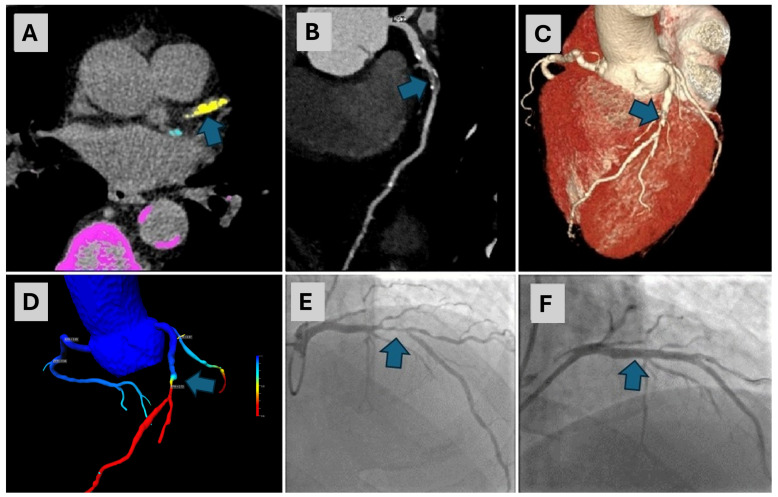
An 80-year-old male with a CAC score of 868.5, exhibiting prominent calcifications in the LAD artery ((A)—arrow), underwent CCTA. The CCTA revealed severe stenosis in the mid-segment of the LAD artery ((B)—arrow, (C)—arrow). A cFFR test confirmed a significant stenosis in the same segment ((D)—arrow), with a cFFR value of less than 0.7. Consequently, ICA was performed, which confirmed 80% stenosis in the LAD ((E)—arrow). This was followed by percutaneous coronary intervention (PCI) with stenting and the final angiography exam showed complete revascularization ((F)—arrow). CAC—coronary artery calcium, LAD—left anterior descending, CCTA—coronary computed tomographic angiography, cFFR—computed fractional flow reserve, ICA—invasive coronary angiography, PCI—percutaneous coronary intervention.
